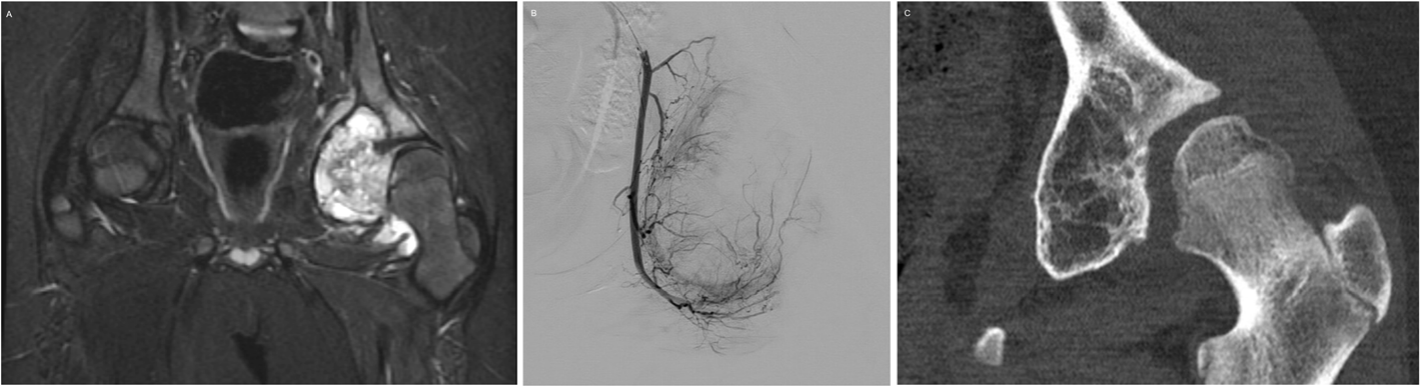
a Short tau inversion recovery (STIR) weighted coronal MR image demonstrating an expansile aneurysmal bone cyst of the left acetabulum in a 10-year-old male. b Digital subtraction angiography demonstrating arterial tumor blush from branches of the obturator artery. These feeders were embolized with 25% N-butyl cyanoacrylate and Lipiodol mixture. c Follow-up coronal CT image obtained 24-months after embolization demonstrating decrease in size of the ABC with ossification
